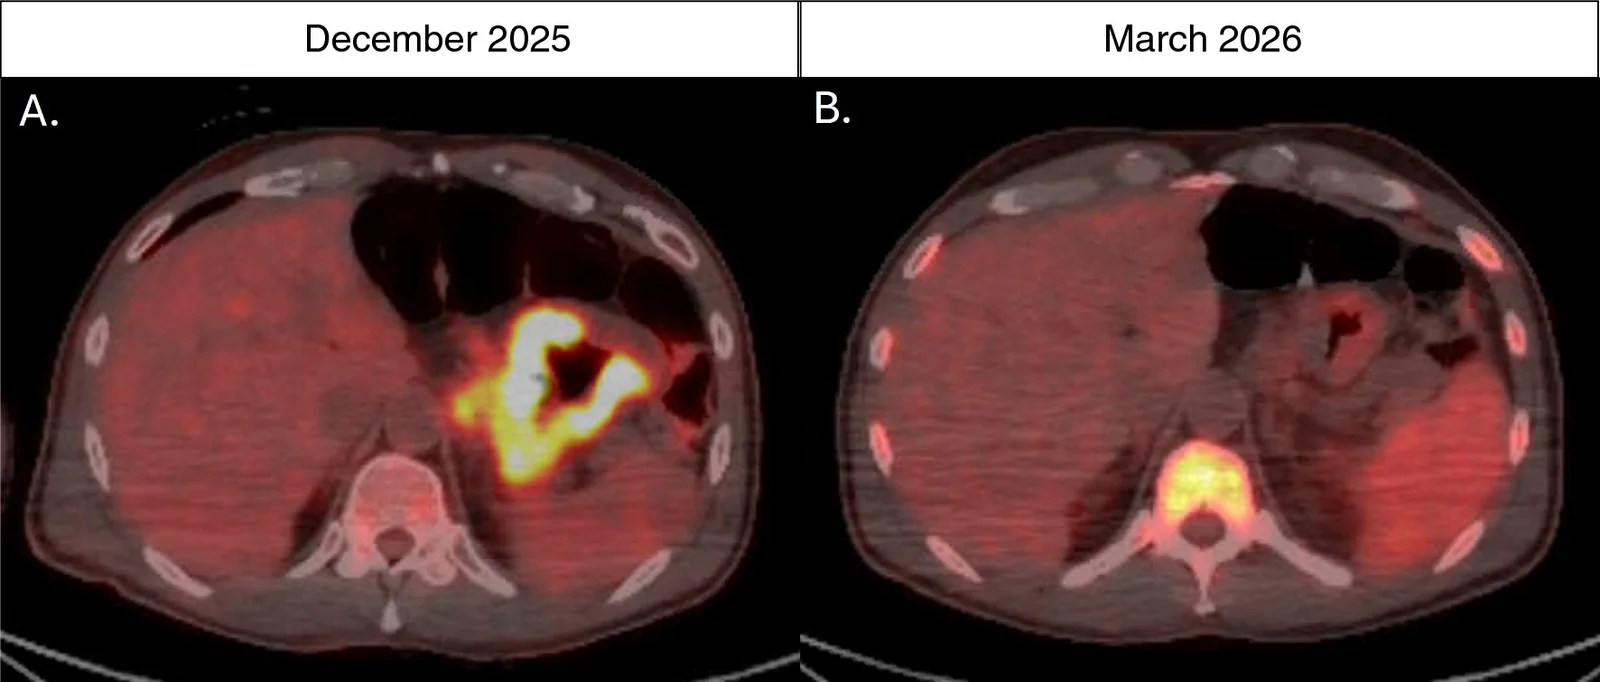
Pre-treatment (A) PET/CT scan from a 51-year-old man who presented with poorly-differentiated gastric adenocarcinoma with linitis plastica. He received FLOT + durvalumab and had a marked clinical response but remained with gastric wall thickening and surround adenopathy on post-treatment PET (B)
